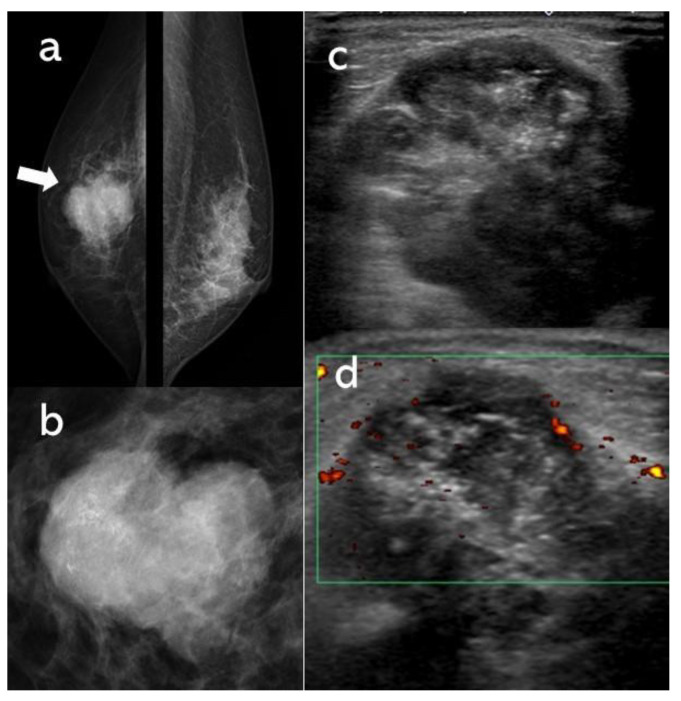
Bilateral mediolateral oblique (a: arrow) and right craniocaudal spot compression (b) mammograms demonstrate a 40-mm lobulated mass with relatively smooth borders containing coarse calcification. Ultrasound B-mode imaging (c) reveals a hypoechoic 40-mm lobulated right breast mass with relatively smooth borders containing a punctate-to-coarse hyperechoic area that corresponds to the calcification and the power Doppler imaging (d) showing blood flow mainly in the peripheral area of the tumor.
